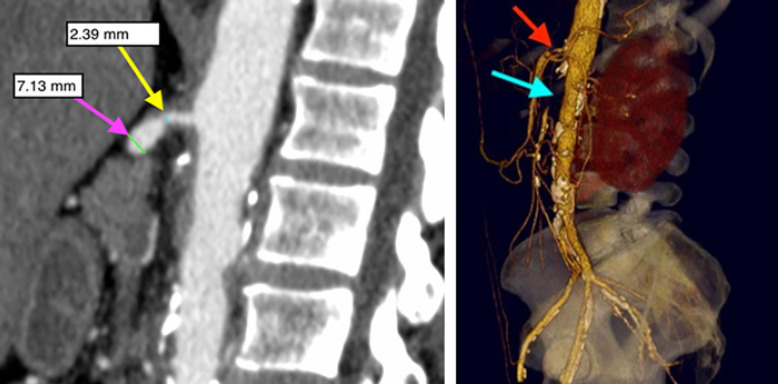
Contrast-enhanced multi-slice CT showing a CT-slice of a sagittal reconstruction in a patient with high-grade stenosis of the CA at its origin (>70% reduction of arterial diameter) and occlusion of the proximal SMA (left) and a 3-D reconstruction of the same patient (right) − red arrow represents the CA-stenosis, and light blue arrow represents the SMA-occlusion (from the Department of Radiology and Nuclear Medicine, University Hospital of Magdeburg [Germany]). SMA, superior mesenteric artery.
